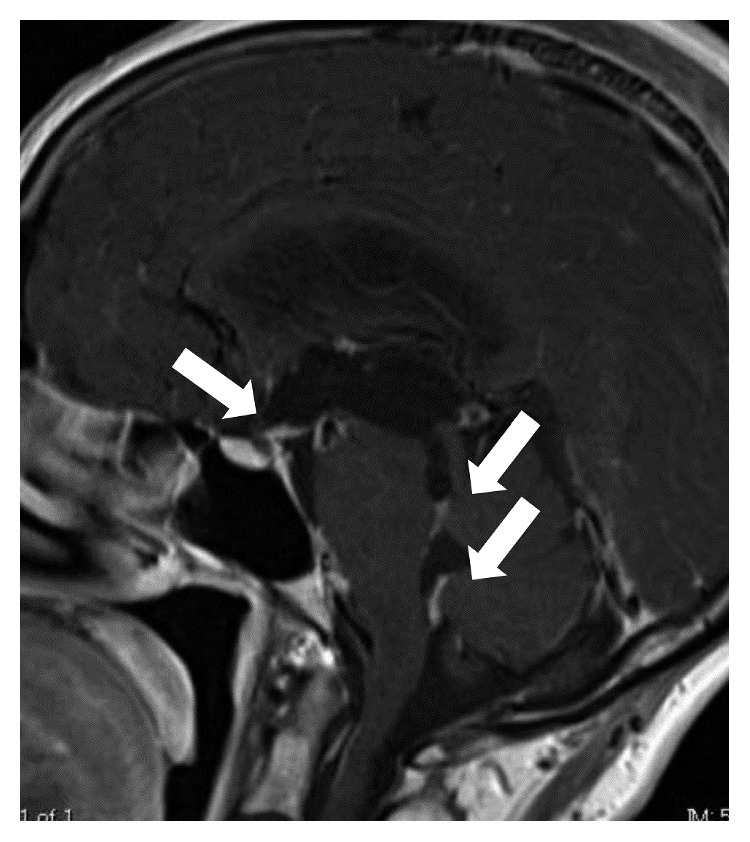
Head MRI showing contrast-enhanced nodular lesions on the floor of the third ventricle, aqueduct of the midbrain, and fourth ventricle.
